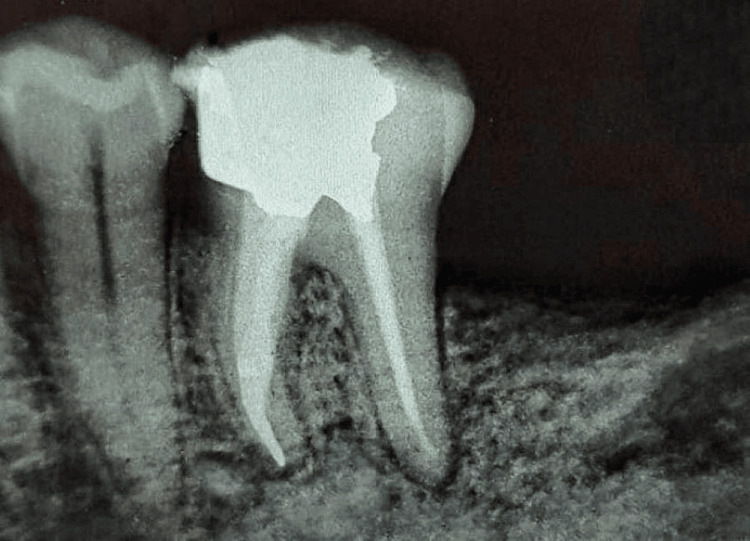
One-month follow-up radiograph A follow-up radiograph reveals the absence of any periapical lesion in the root canal-treated tooth, showing signs of healing.
